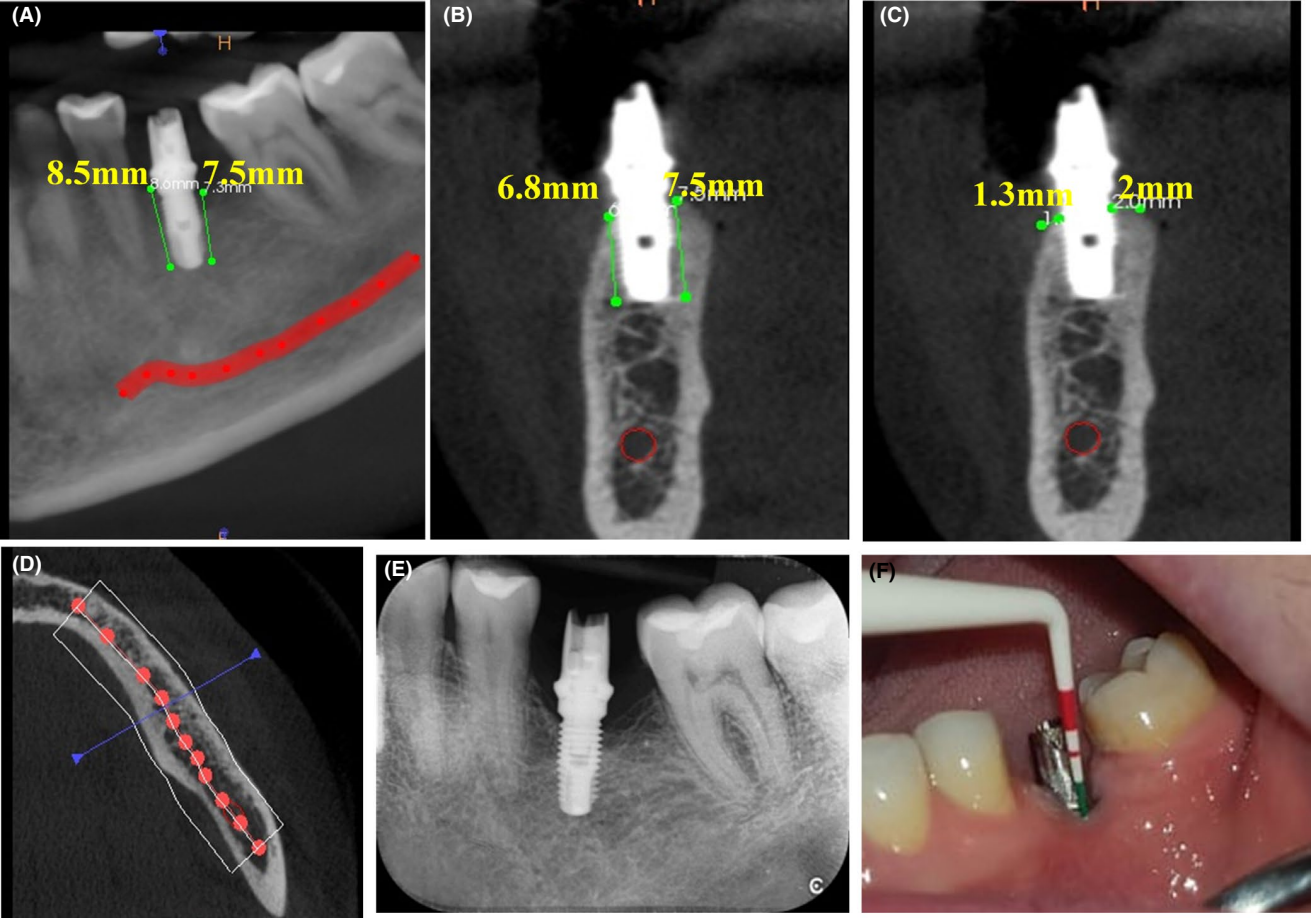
The CBCT imaging of a peri‐implantitis case showing bone healing in mesiodistal view (A), buccolingual view (B,C) and axial view (D) around dental implant, Intraoral periapical radiograph of implant site (E), pocket depth around the implant (F) 1 y after treatment
